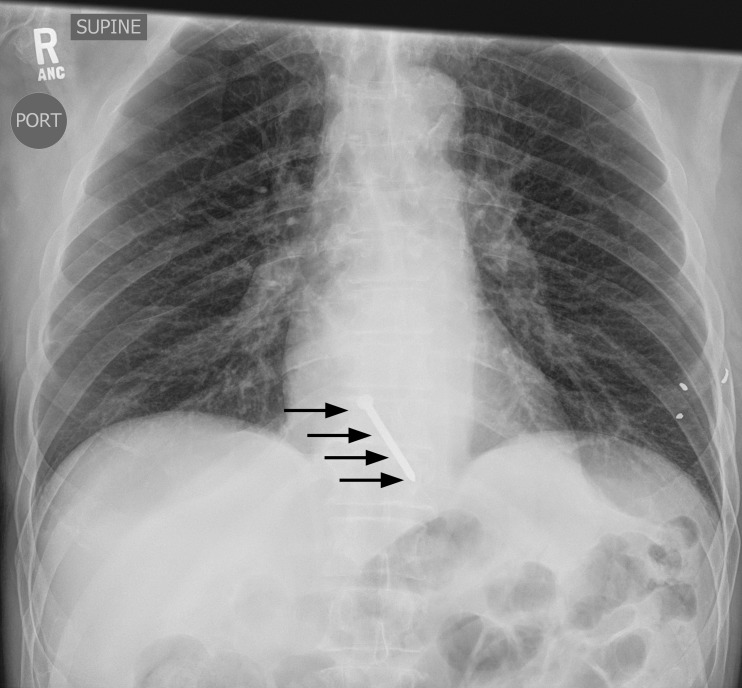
Anteroposterior Chest X-Ray Anteroposterior chest X-ray showing metallic nail overlying the heart (arrows). Also seen are buckshot overlying the left lateral chest wall and a healed left lateral rib fracture associated with a prior injury.
